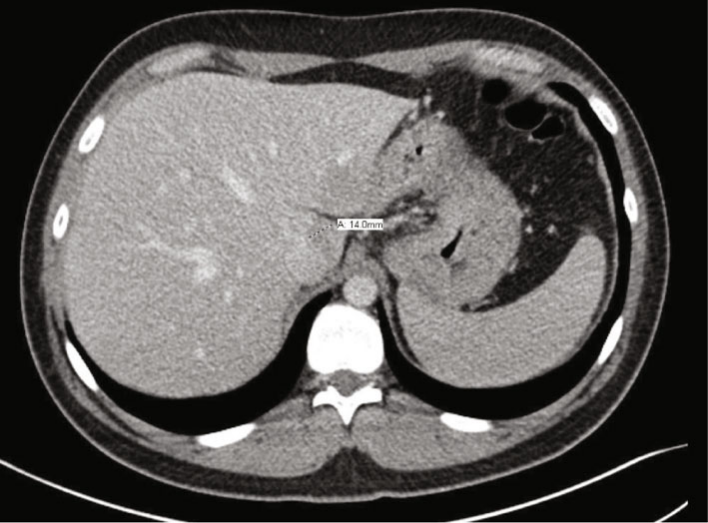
CT of the abdomen: significant regression of the metastatic nodular lesion to 1.4 cm in the pancreatic neck following radiation therapy.
